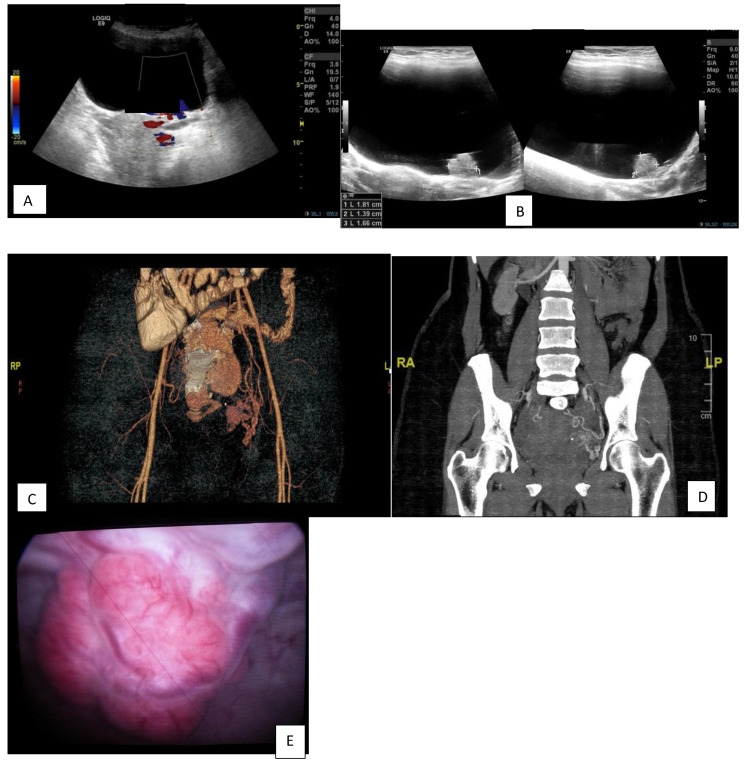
Lesion arising from the urinary bladder wall and diagnostic cystoscopy. (A): An ultrasound shows a Pepsi sign on the color Doppler. (B): an ultrasound grey scale shows a lesion from the urinary bladder wall. (C,D): Ct with Iv contrast and processed image of the CT shows a hyper-vascular lesion in the urinary bladder wall. (E): a large, pulsatile, submucosal mass and a wide-based stalk in Diagnostic Cystoscopy.
